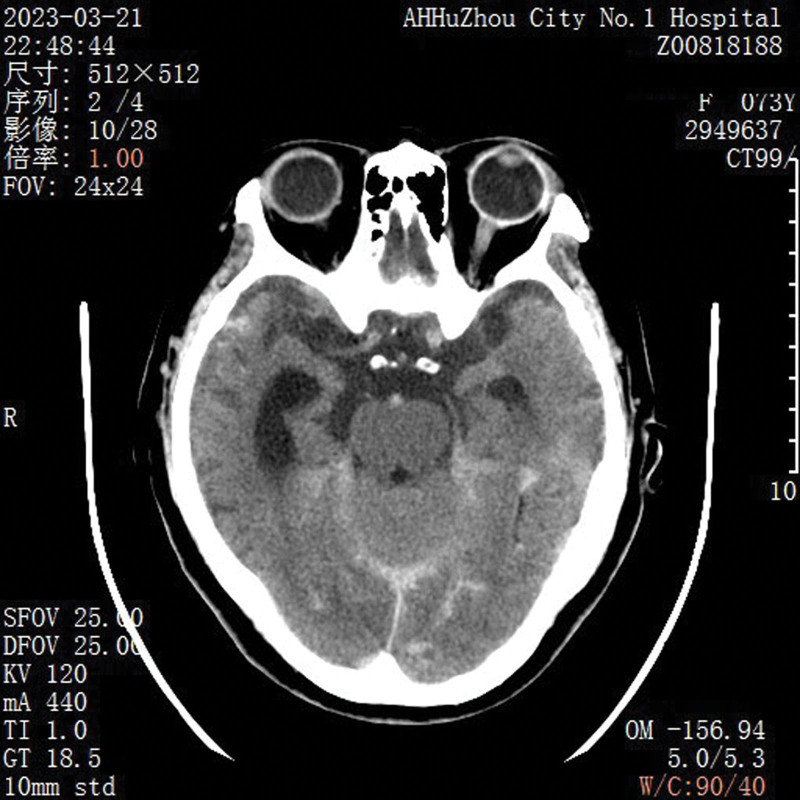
CT images after the procedure were completed and there was no evidence of bleeding. CT = computed tomography.
